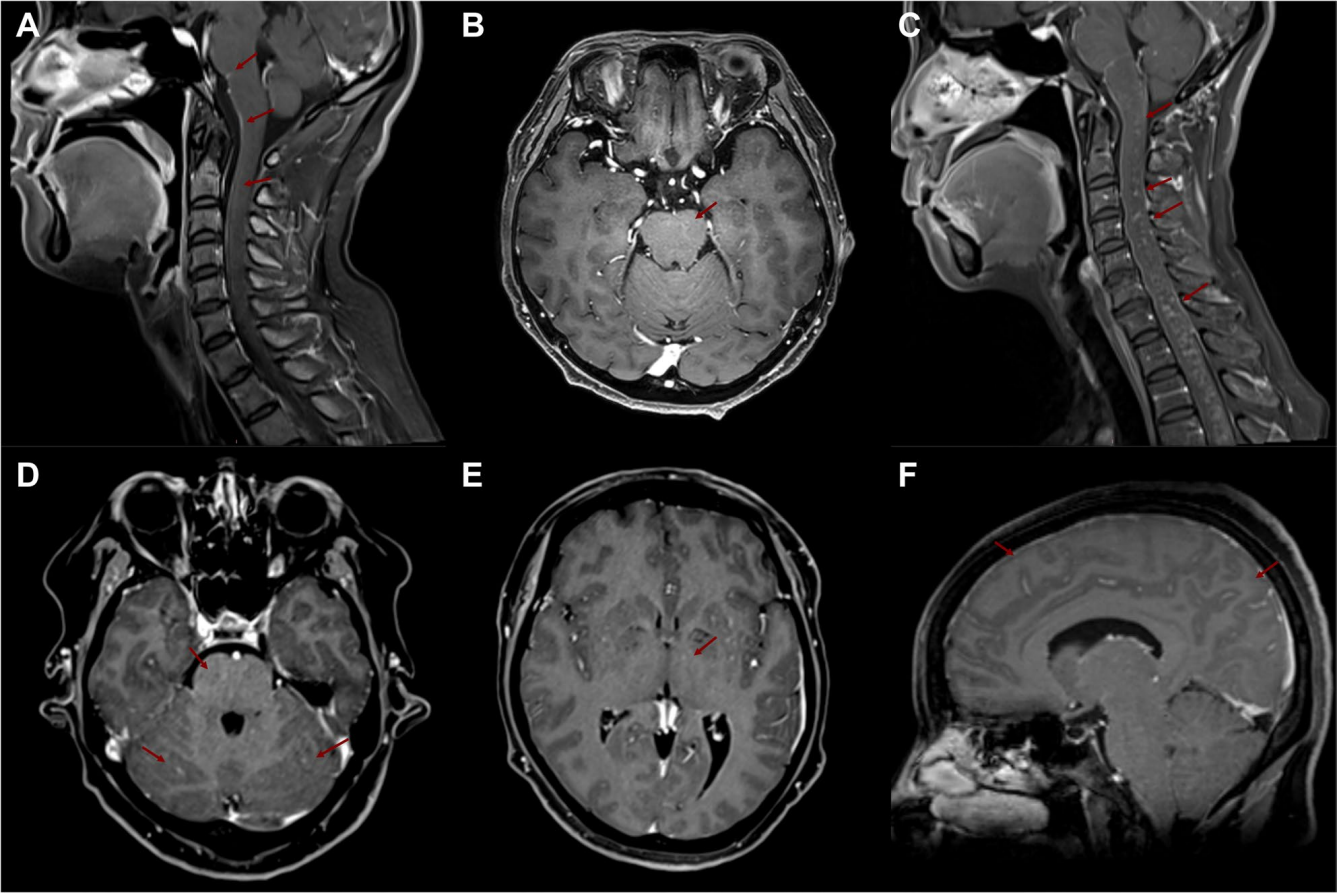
Brain and spinal cord MRI findings in three GFAP-A patients with abnormal imaging. A Patient 2: T1-weighted contrast-enhanced image showing leptomeningeal enhancement along the brainstem and ventral surface of the spinal cord (arrows). B, C Patient 3: Axial and sagittal T1-weighted contrast-enhanced images demonstrating multiple punctate and patchy enhancements within the brainstem and cervical spinal cord (arrows). D, E, F Patient 6: T1-weighted contrast-enhanced images revealing punctate and linear enhancements in the corona radiata, basal ganglia, brainstem, and cerebellum, accompanied by diffuse leptomeningeal enhancement along the cerebral sulci (arrows)
